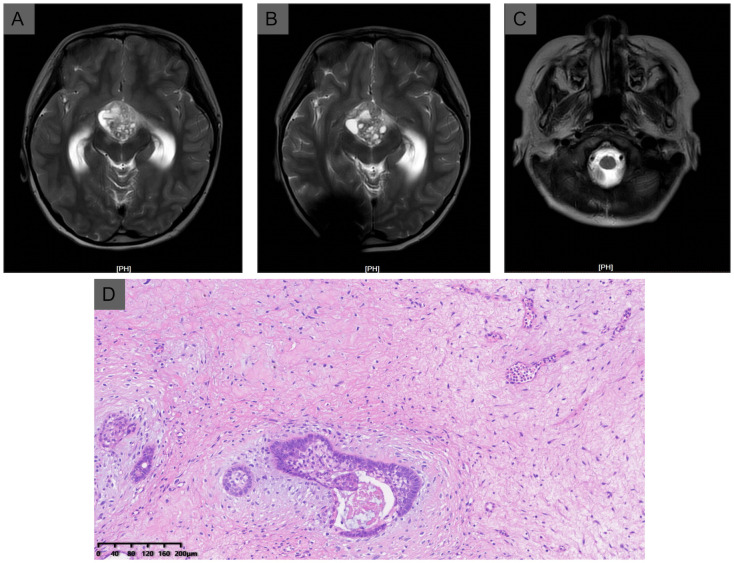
MRI findings and pathology in Case 1. (A) Initial MRI showing a heterogeneous suprasellar mass with cystic components, pituitary involvement, and compression of the optic pathway. (B) Post-treatment MRI revealing interval enlargement of the mass despite a biochemical response. (C) Postoperative MRI after gross total resection showing no residual tumor. (D) Pathological examination after surgical resection confirming mature teratoma without malignant elements.
